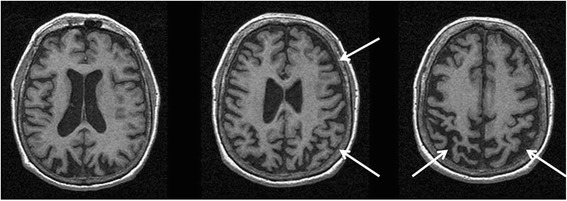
MRI of a patient with a pathological diagnosis of Corticobasal Degeneration. Serial axial T1 sequences showing right greater than left parietofrontal atrophy typical of that seen in Corticobasal Syndrome. In this case, the patient had a confirmed pathological diagnosis of Corticobasal Degeneration
